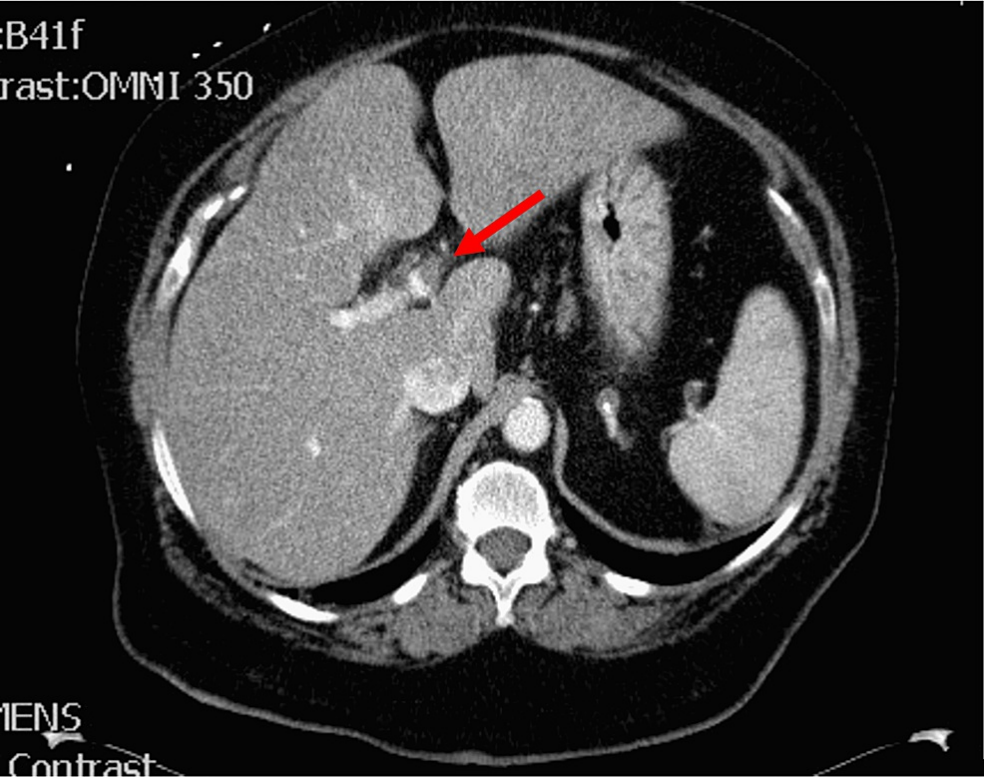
CT scan showing a bland thrombus within the left portal vein with subsequent mild heterogenous enhancement of the left hepatic lobe
